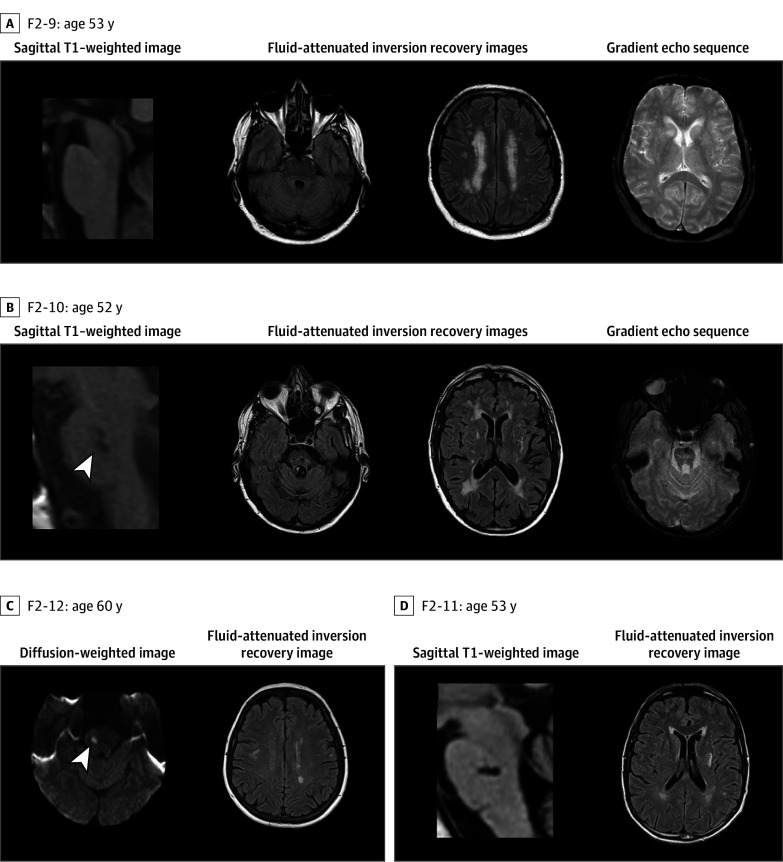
Magnetic Resonance Imaging Data of Patients From Family F2 The first column presents sagittal T1–weighted images except for C (patient F2-12), which presents diffusion-weighted images. Fluid-attenuated inversion recovery images are presented in columns 2 to 4, except for A (patient F2-9) and B (patient F2-10) in column 4, which present gradient echo sequences, and D (patient F2-11) in column 3, which presents sagittal T1 images. All patients except patient F2-9 (A) present pontine infarcts and vascular leukoencephalopathy associated with hemispheric lacunes.
